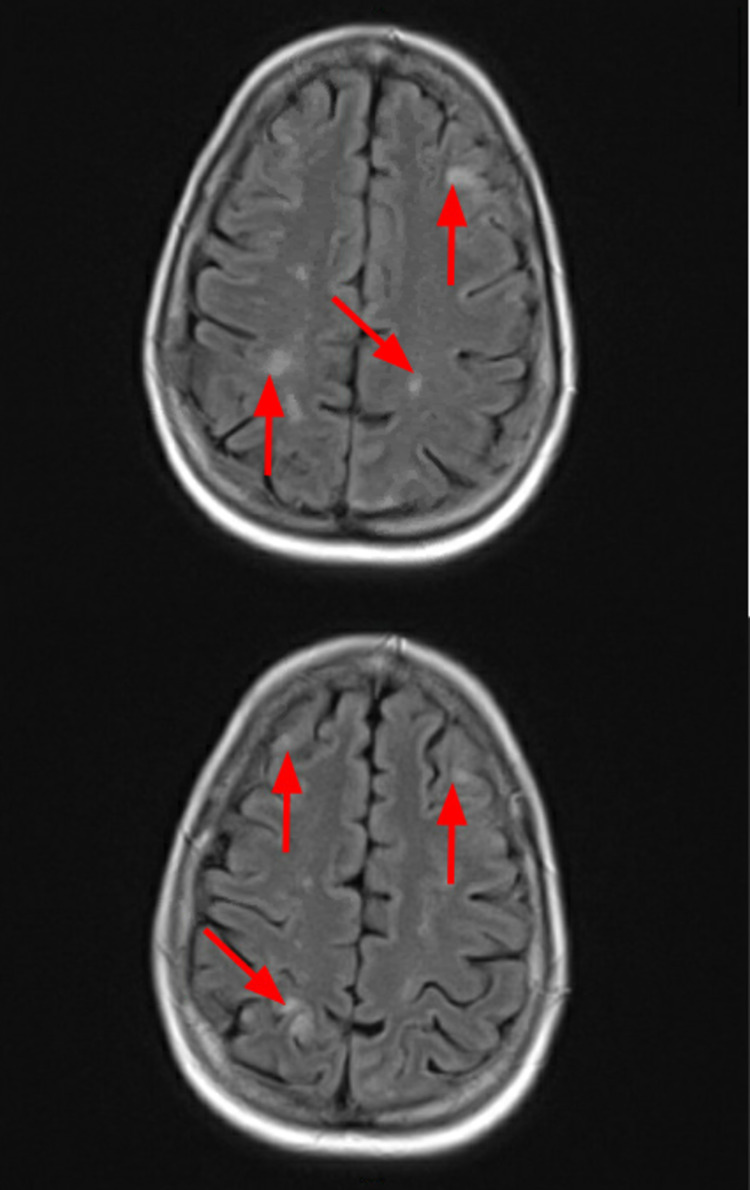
MRI brain images showing multiple lesions (arrows) in the cortex of the brain.
